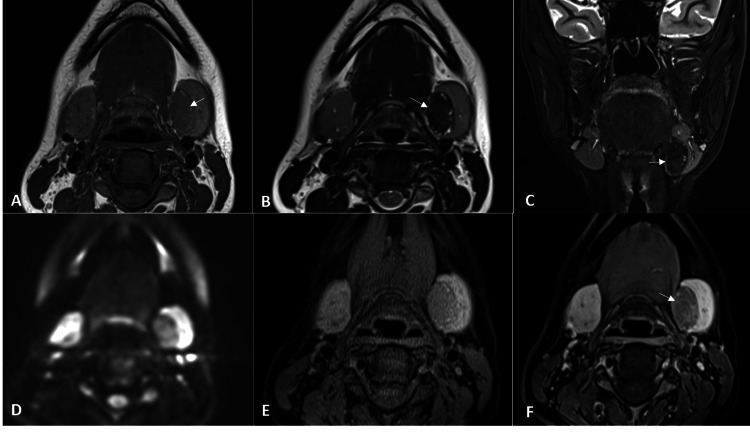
Magnetic resonance imaging (MRI) of the neck showing a well-defined, oval lesion (white arrows) in left submandibular space, appearing isointense to slightly hyperintense to muscle on T1-weighted (A) and T2-weighted images (B) and STIR images (C) with no diffusion restriction (D). The lesion showed mild to moderate enhancement on post gadolinium T1 FS images (E, F).
